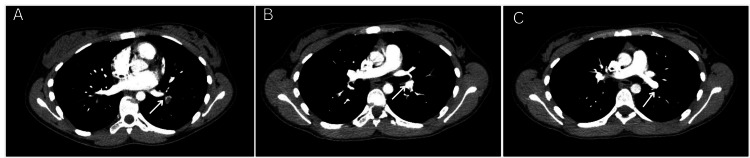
Axial CT pulmonary angiography (CTPA) images (A, B, and C) from Case 1 demonstrating multiple filling defects within proximal segmental pulmonary artery branches supplying the left lower lung lobe (arrows)
